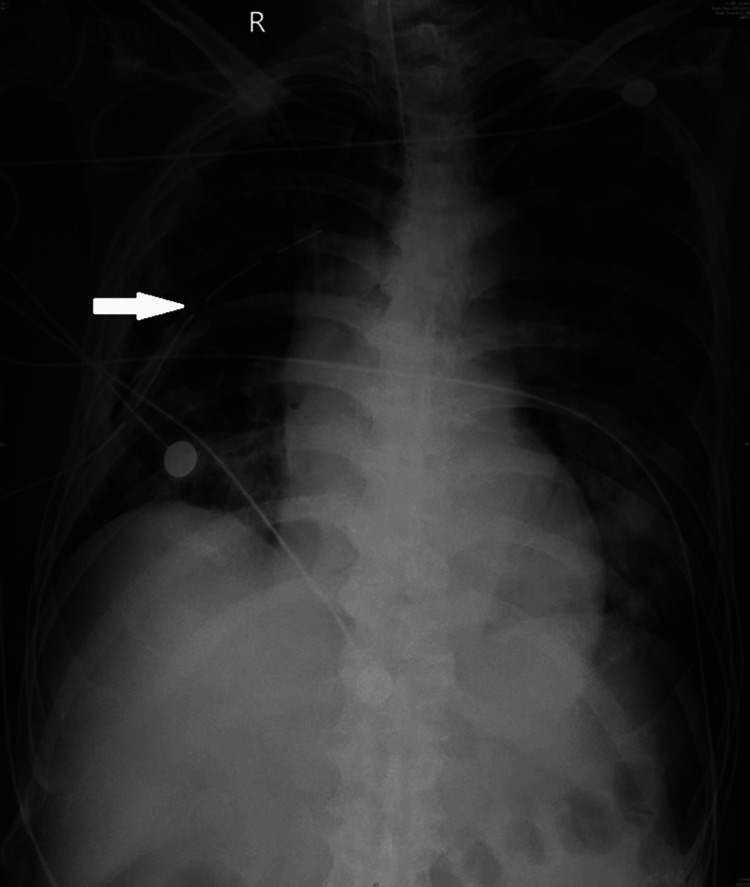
Mid-treatment Portable Chest X-ray An anteroposterior view shows the right-sided thoracic drainage tube in situ (white arrow). There is interval reduction in the right pleural opacity compared with the initial CT scan, consistent with partial resolution of the pyopneumothorax.
